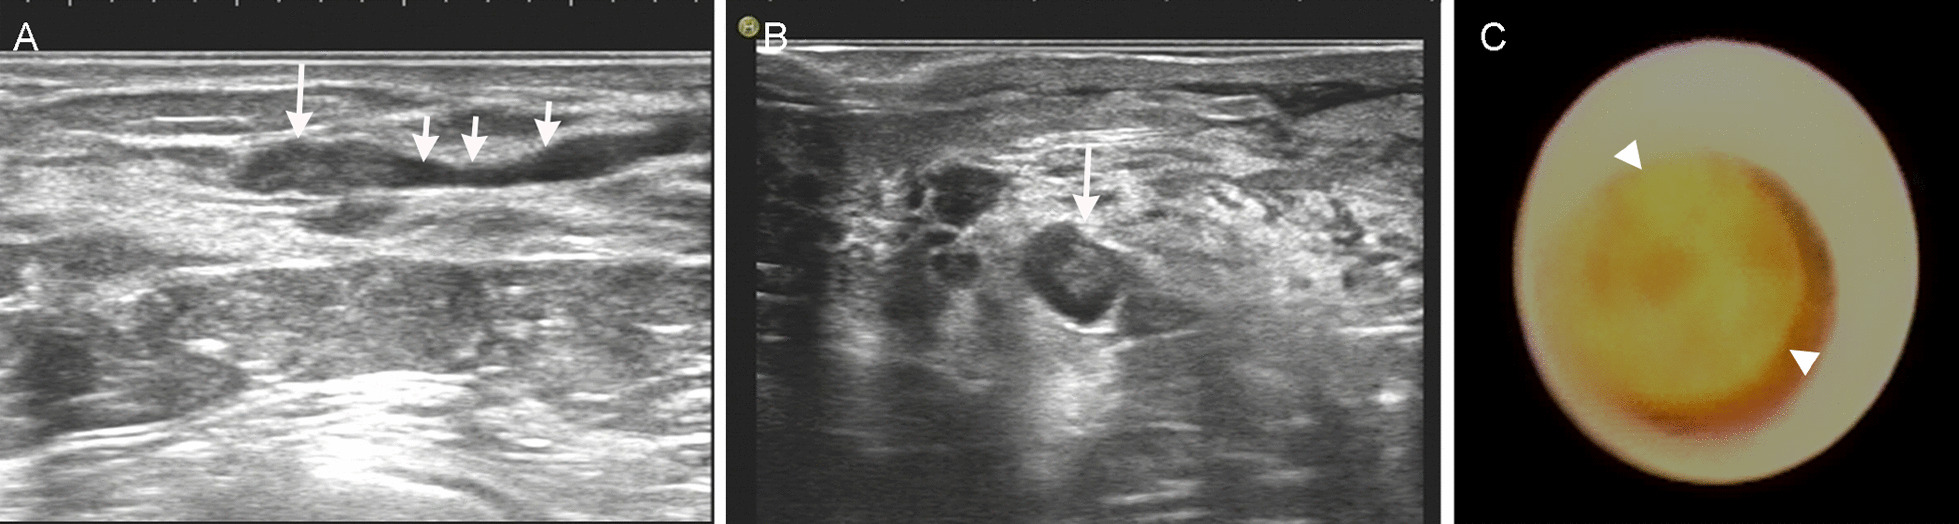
A 45-year-old female patient with yellow nipple discharge for 1 month. Comparison of ultrasound sonogram and fiberoptic ductoscopy for intraductal papilloma was performed. Sections from A the long and B the short axes in conventional two-dimensional ultrasound revealed local ductal dilation (short arrow) in the 3 o'clock direction in the right breast, with solid isoechoic nodular filling (long arrow) in the distal lumen of the dilated segment. Fiberoptic ductoscopy C showing dilation of the breast duct with smooth ductal walls and one light yellow wart-like neoplasm obstructing the lumen
